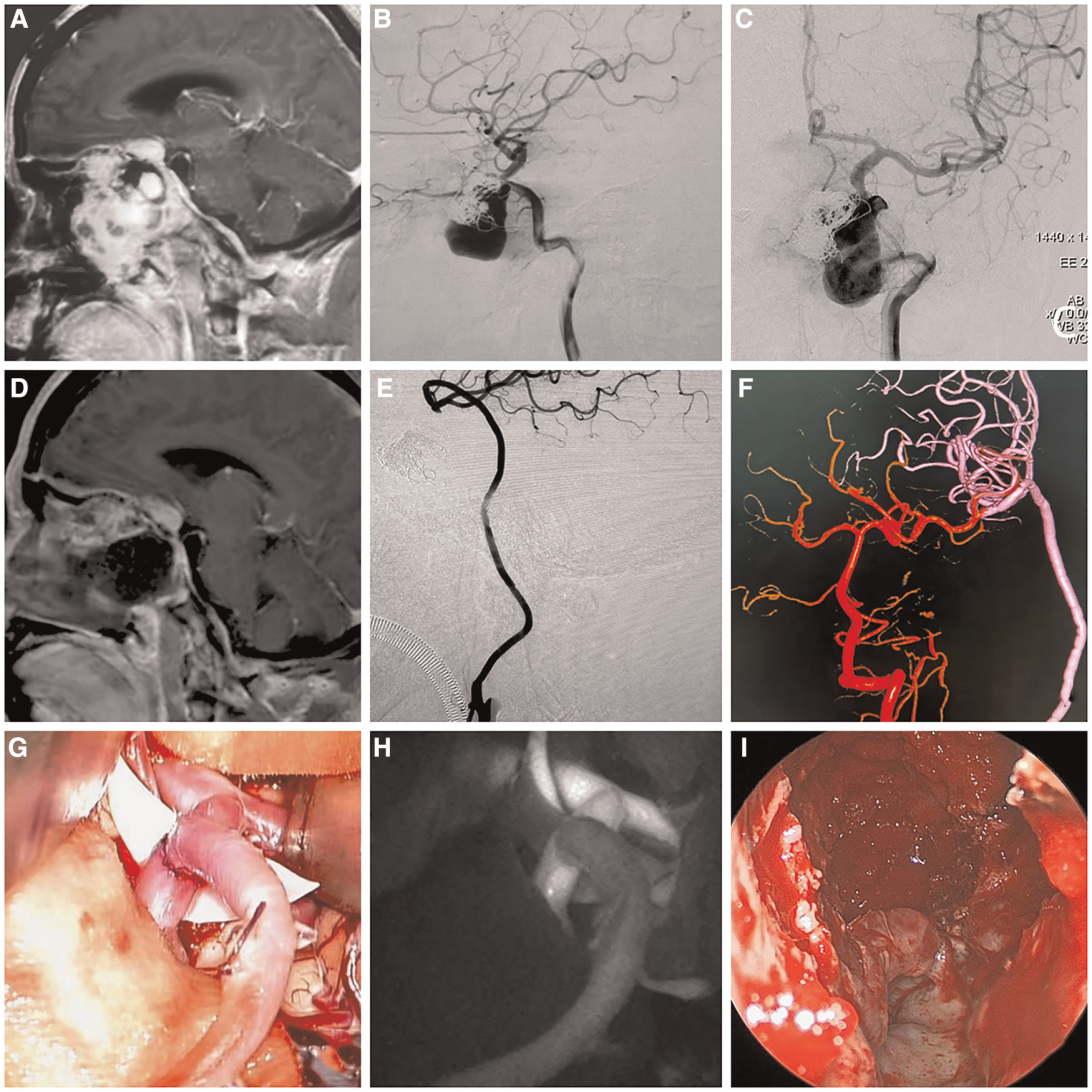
Images of a patient with recurrent fibrous dysplasia combined with internal carotid artery aneurysm who received Fukushima bypass type III (ECA-RA-M2) combined with transnasal endoscopic surgery to remove the affected bone, achieved optic nerve decompression and resection of the left internal carotid artery aneurysm. (A) Preoperative enhanced cranial magnetic resonance imaging (MRI) showing the internal carotid artery was invaded, and an aneurysm was formed. (B, C) Preoperative lateral and front digital subtraction angiography (DSA) projections showing the internal carotid artery and aneurysm. (D) Postoperative enhanced cranial MRI showing subtotal resection of the tumor and adequate decompression. (E, F) Postoperative cerebral DSA showing good patency of the radial artery graft, no visualization of ICA-C1 to ICA-C4 segments, and anterior cerebral circulation blood supply from posterior cerebral circulation. (G) Intraoperative image of Fukushima bypass type III (ECA-RA-M2). (H) Intraoperative fluorescein angiography showing good patency of the radial artery graft. (I) Intraoperative images of endoscopic subtotal resection of tumors.
